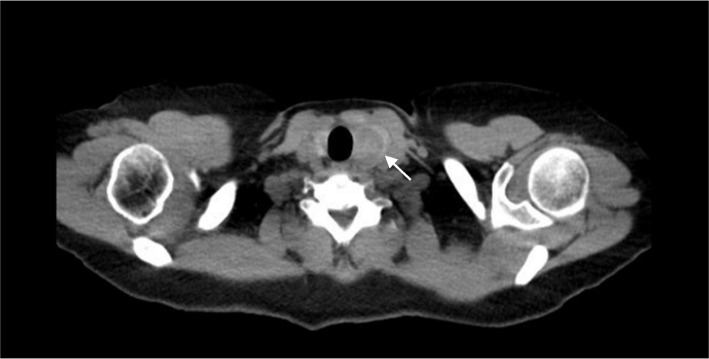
CT chest axial view. Arrow denotes 2.6 cm  left thyroid nodule
